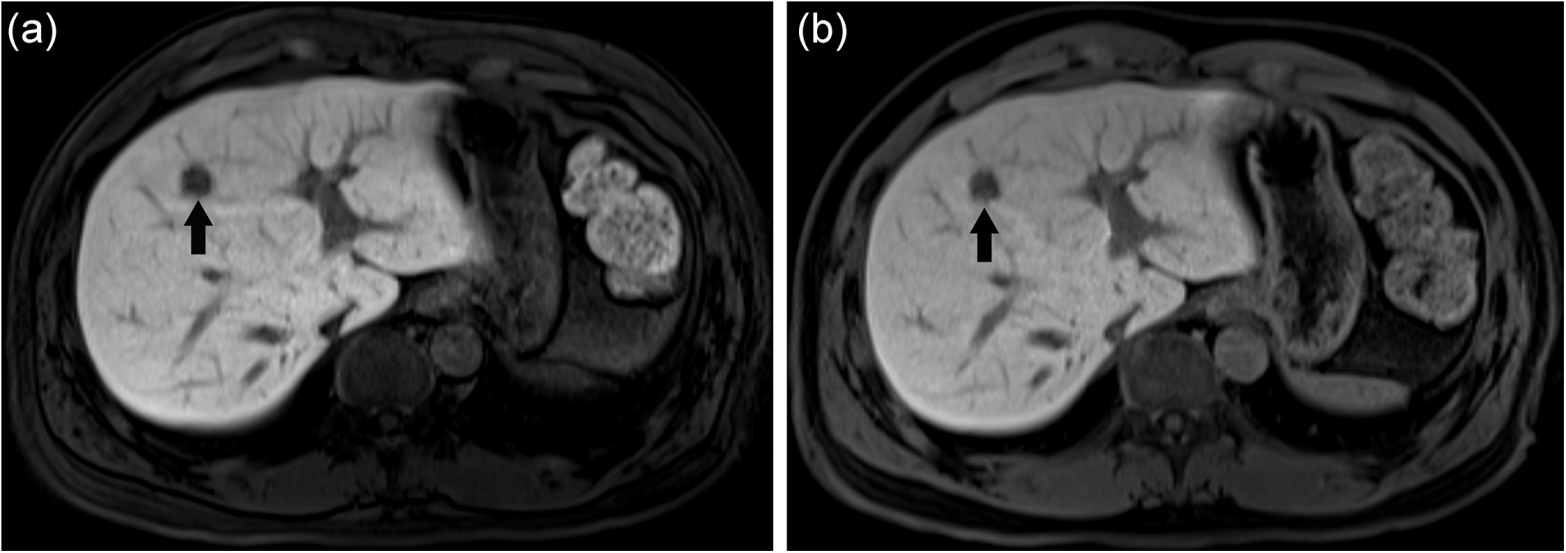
43-year-old male with pathologically proven liver metastasis from rectal cancer. Gadoxetic acid-enhanced T1-weighted MR hepatocyte phase images taken with a 10-minute delay and a 30° flip angle (10min-FA30) (a) and a 20-minute delay and a 10° flip angle (20min-FA10) (b). The liver metastasis in segment 4 showed low signal intensity related to liver parenchyma on both images (dark arrows). The lesion-to-liver CNR of the 10min-FA30 image (190.3) was superior to that of the 20min-FA10 image (137.3). Two different radiologists each gave the 10min-FA30 and 20min-FA10 images a mean subjective score for focal hepatic lesion presence of 4.0.
